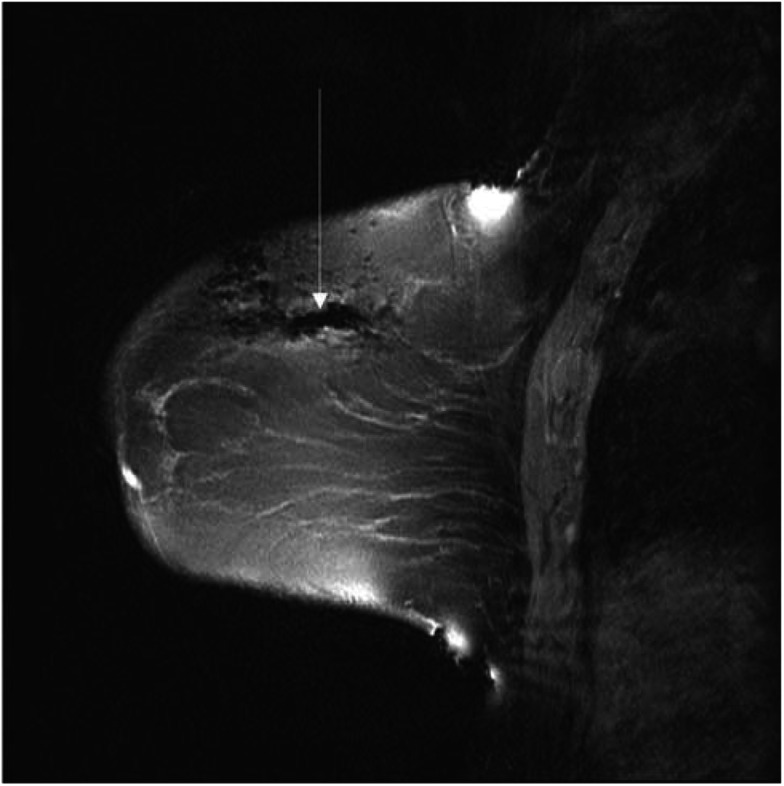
T1-weighted postcontrast fat-suppressed image of a breast showing signal voids from surgical instrument metal fragments.
